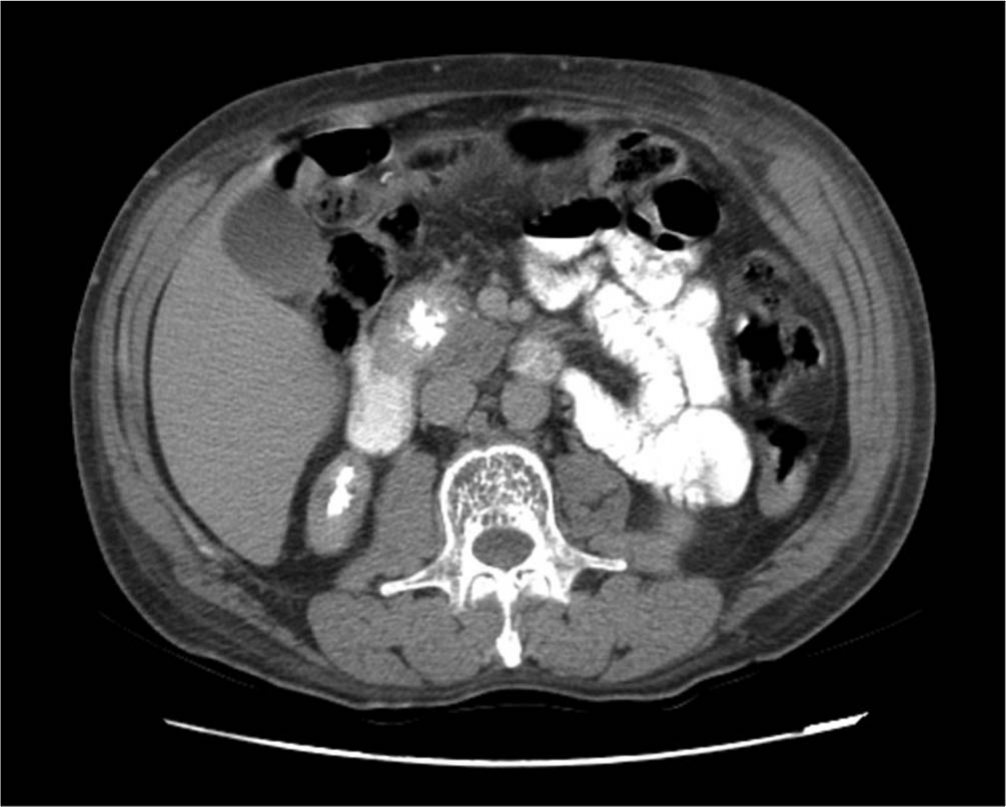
Triphasic computed tomography of the pancreas, late phase, showing a tumor in the head of the pancreas.
